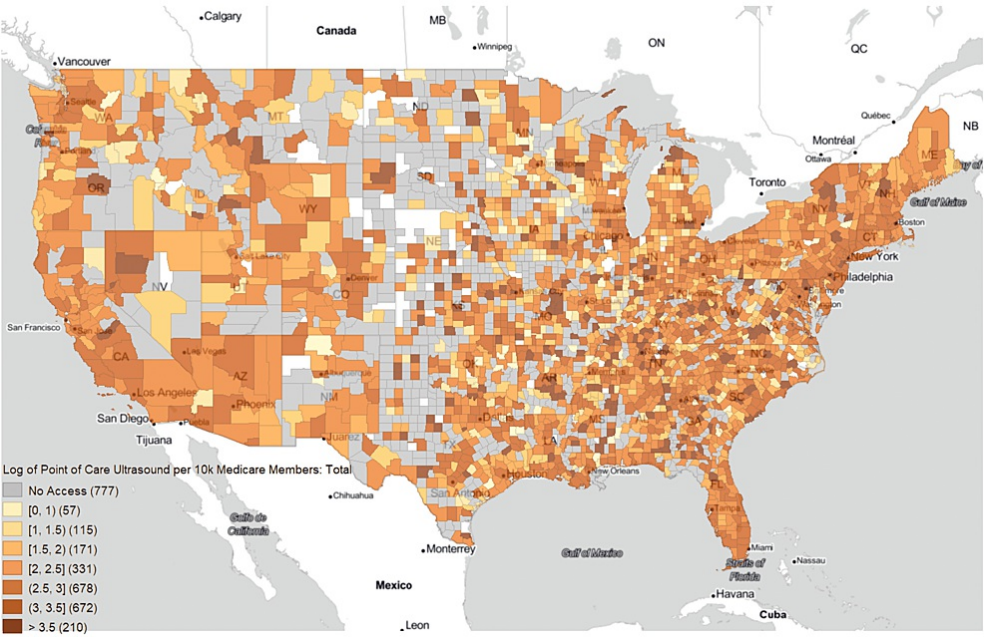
Choropleth map of log-scaled average yearly point-of-care ultrasound uses per 10k Medicare members Counties without access are in gray. Counties excluded from analysis are in white.
